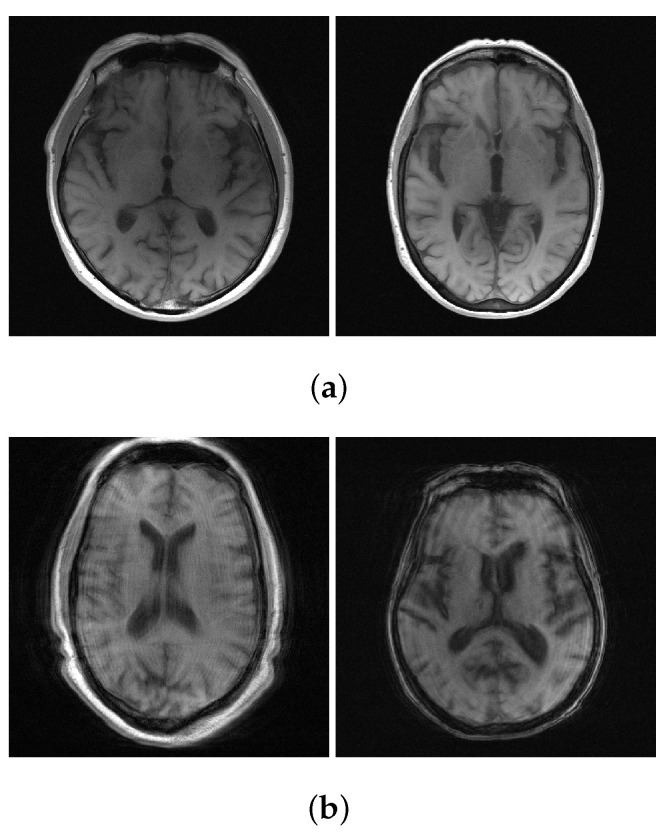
Examples of the NYU fastMRI brain dataset. (a) Artifact-free T1 weighted MR image; (b) Ringing artifact T1 weighted MR image.
